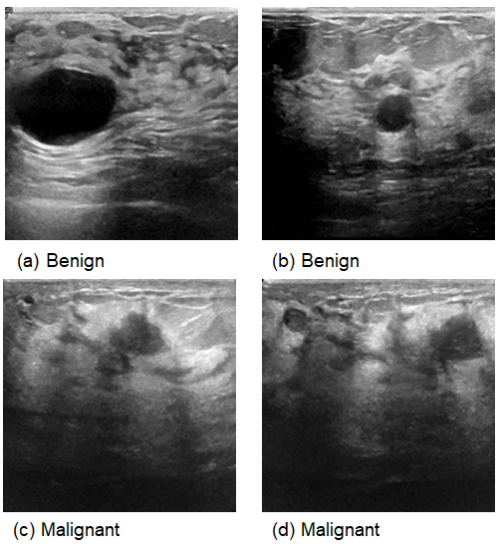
Samples from Ultrasound images.
